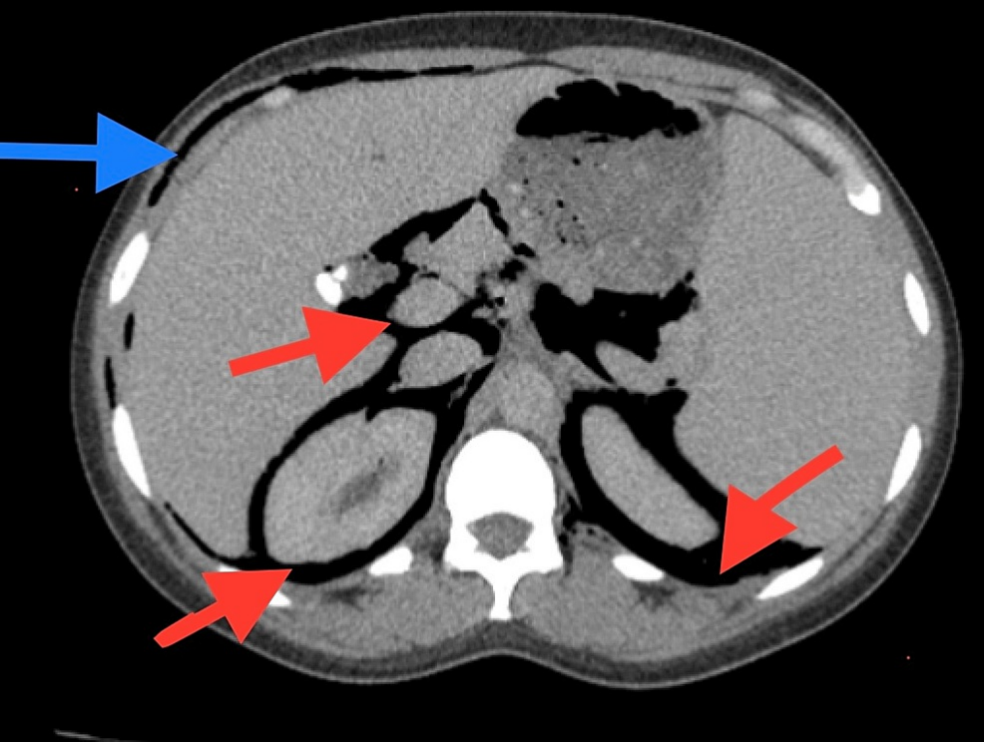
Computed tomography of the abdomen/pelvis with intravenous contrast showing subcutaneous emphysema (blue arrow) and pneumoperitoneum/retroperitoneum (red arrows)
